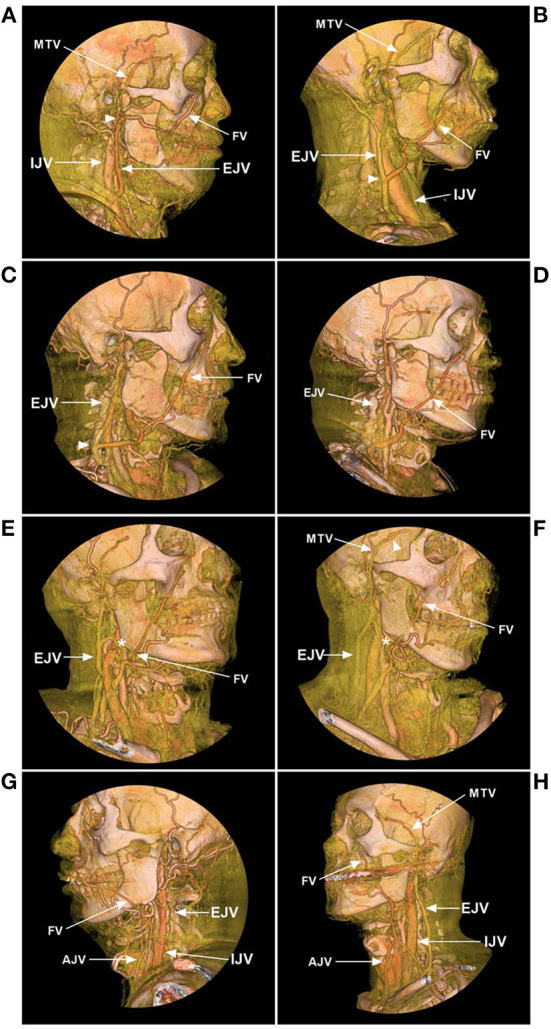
FV variance of CTA. (A) Lateral view CTA shows that the FV courses posteriorly and combines with the MTV at the mandibular joint (arrowhead) from the EJV. (B) Lateral view CTA shows that the FV descends obliquely and curves around the inferior edge of the mandible and flows into the EJV at the middle cervical region (arrowhead). (C) Lateral view CTA shows that the FV flows into the EJV in the low cervical region (arrowhead). (D) Lateral view CTA shows that the FV descends obliquely and curves around the inferior edge of the mandible and flows into the subclavian vein without connection with the IJV or EJV. (E, F) Oblique view CTAs shows that the FV flows into the subclavian vein with the connection with the EJV (asterisks). (G, H) Oblique view CTAs shows that the FVs mainly flow into the AJVs, with the connection with the EJVs. AJV, anterior jugular vein; CTA, computed tomography angiography; EJV, external jugular vein; FV, facial vein; IJV, internal jugular vein; MTV, middle temporal vein.
